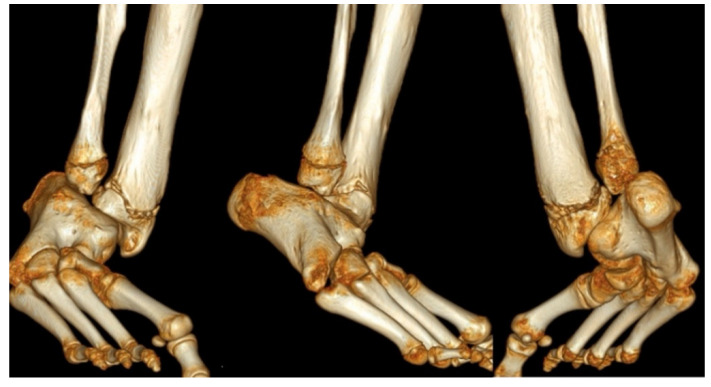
A three-dimensional CT scan of distal tibia and foot (left Anteroposterior (AP) view, middle lateral view and right posteroanterior (PA) views) in a 13-year-old boy with untreated type 3c fibular hemimelia with fixed equino-valgus of the hindfoot. Despite the presence of a fibula, there is valgus-procurvatum malorientation of the ankle plafond, and a malunited subtalar coalition. The calcaneus is articulating with the fibula. There are also calcaneo-cuboid and talo-navicular coalitions with midfoot adductus.
